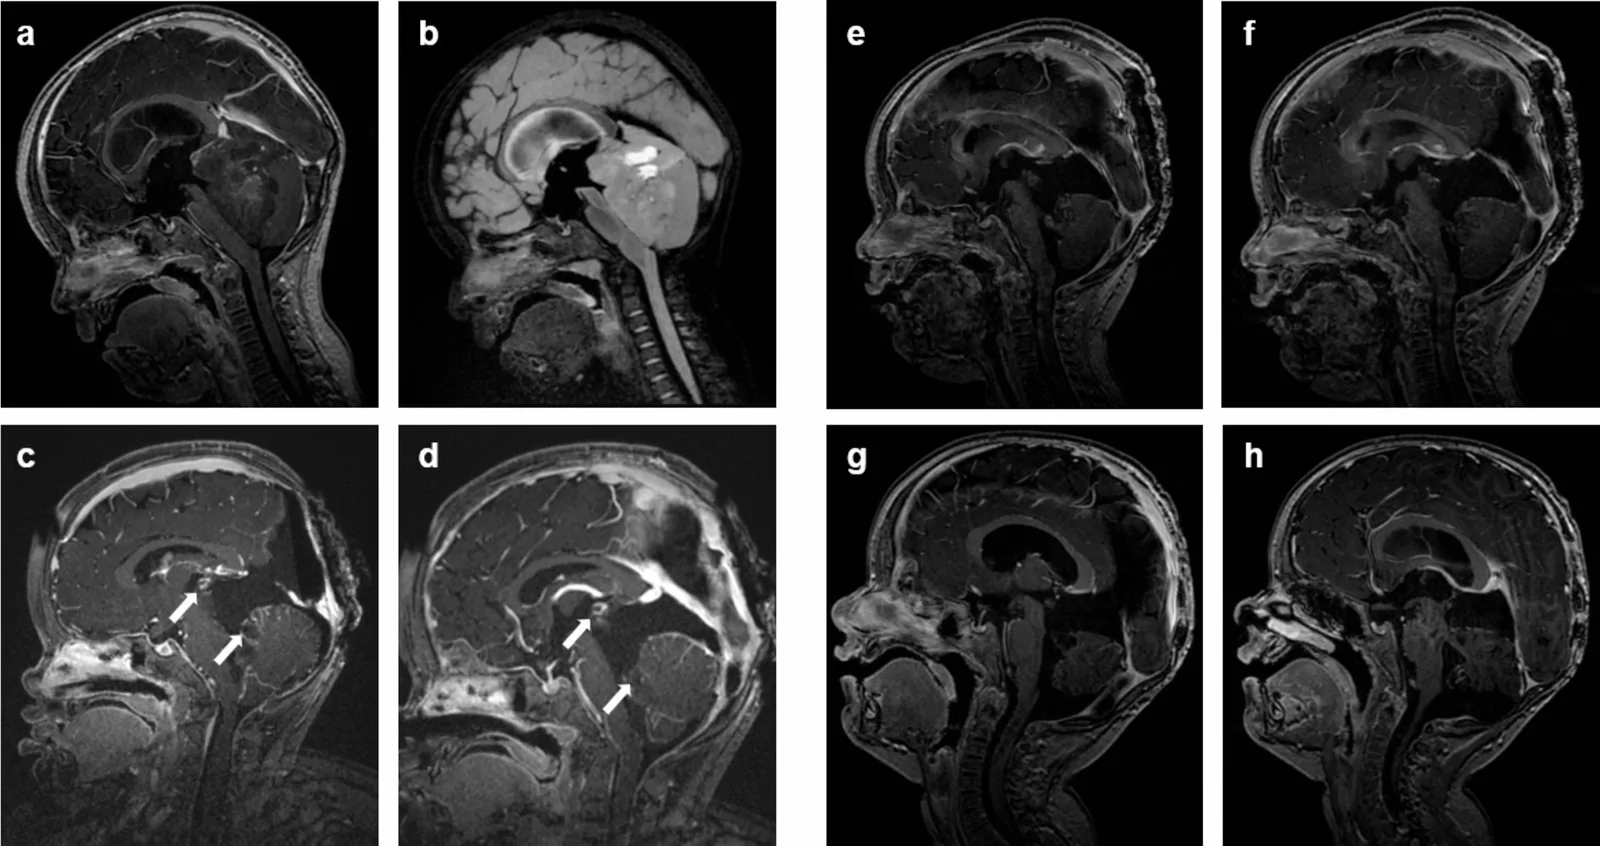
A 1.5-year-old patient presented with an acute onset of ataxia, recurrent falls, and vomiting. Preoperative MRI (T1-weighted with contrast sagittal [a], FLAIR sagittal [b]) revealed a large mass in the cisterna laminae quadrigeminae. Based on the imaging findings, an ATRT was suspected, and the tumor board recommended gross total resection (GTR) as the surgical goal. Intraoperative MRI (c, d) confirmed that the majority of the tumor had been removed but demonstrated multiple small residual tumor nodules (arrow). Additional tumor resection was therefore performed. Postoperative MRI (e, f), obtained the following day, confirmed complete tumor removal resulting in a final extent of resection (EoR) of GTR. The patient subsequently received adjuvant proton radiotherapy according to the EU-RHAB protocol and chemotherapy. Follow-up MRI at 4 months postoperatively showed no evidence of tumor recurrence (g, h)
